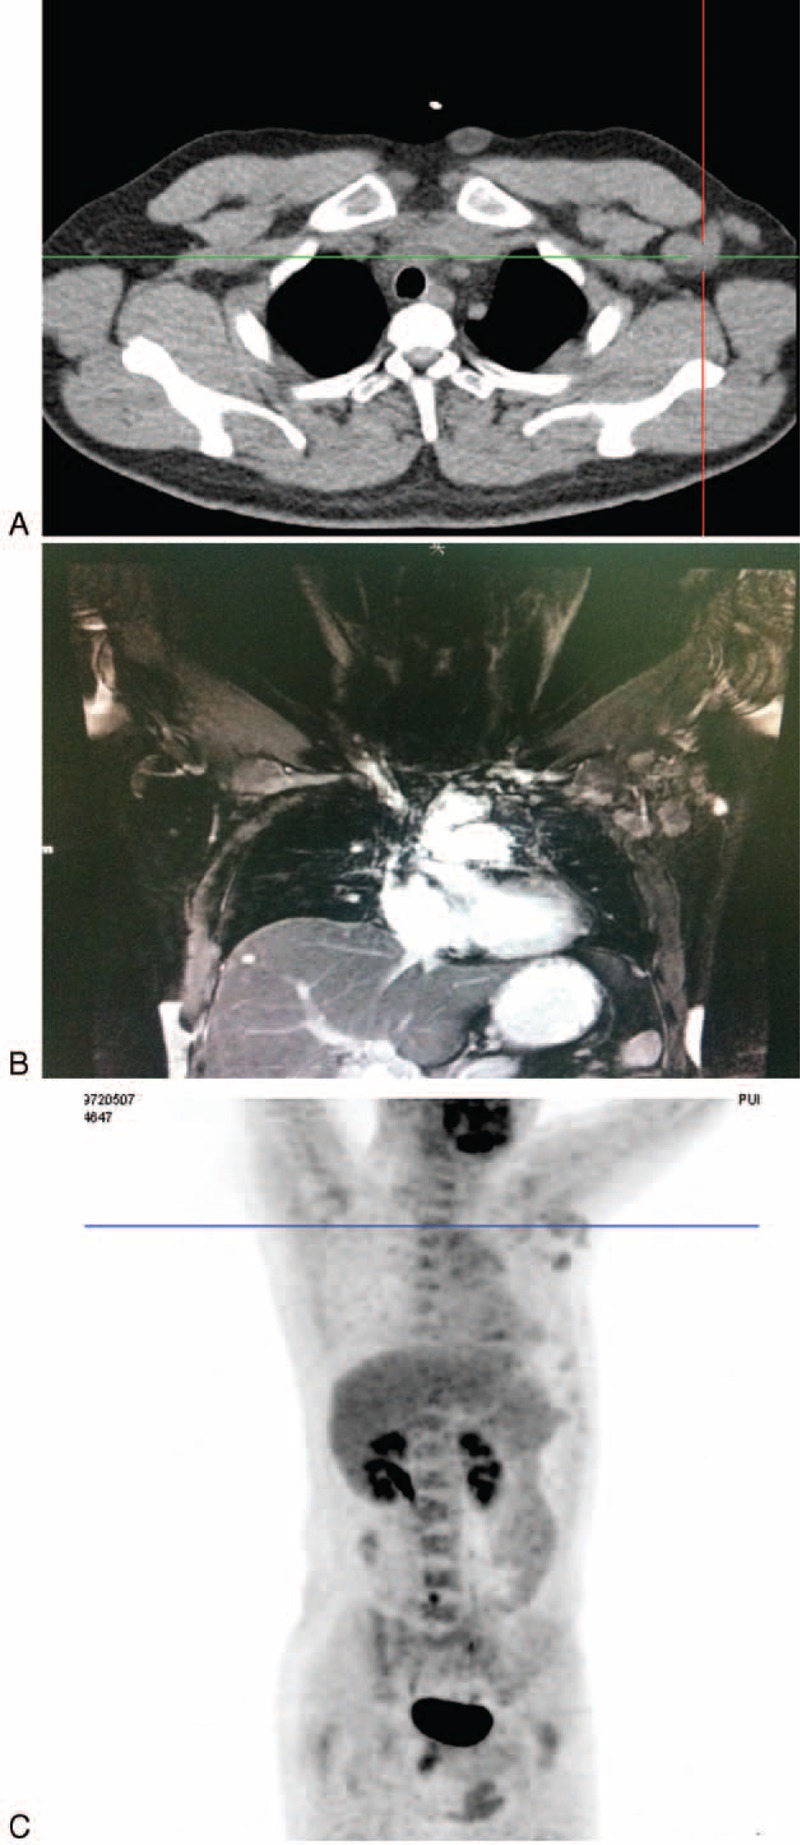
(A) Initial chest CT showing several enlarged left lymph nodes. (B) On magnetic resonance imaging, it manifested as multiple fusional lymph nodes with irregular margins on T2-weighted images. (C) Positron emission tomography/CT showing malignant lymph nodes with focally increased radiotracer activity in left axilla without other suspicious malignant lesions. CT = computed tomography.
